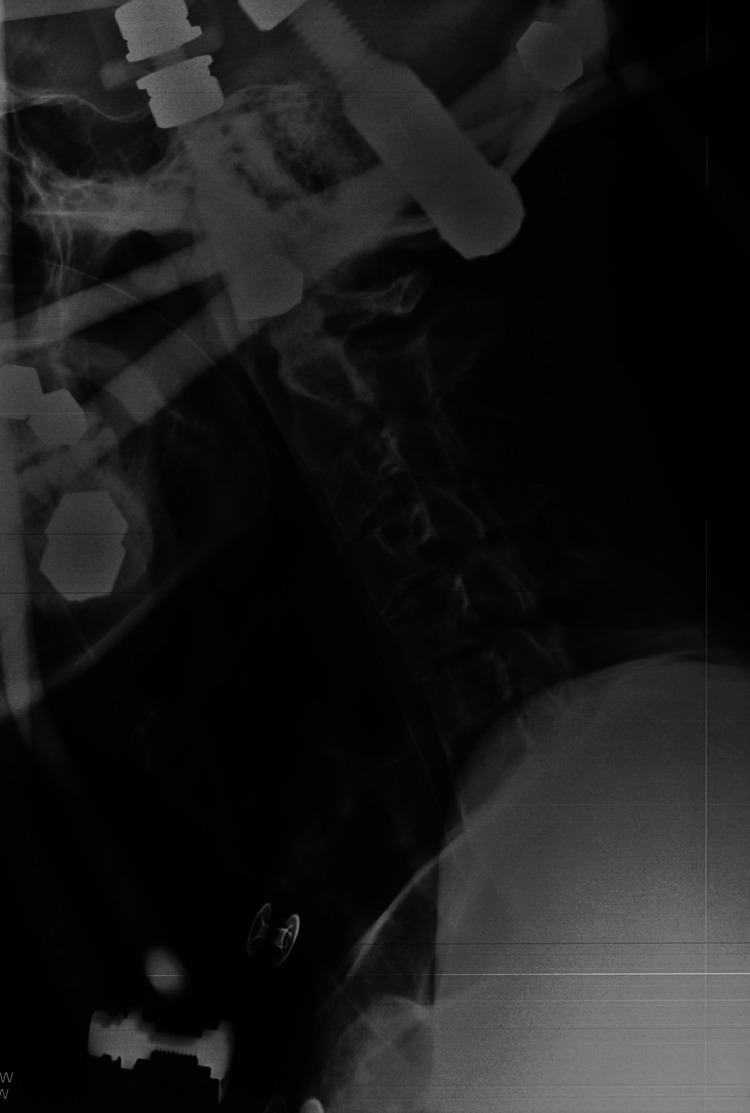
Reduction of cervical fractures in patients with AS from the perspective of a C-arm radiograph system. AS, ankylosing spondylitis.
